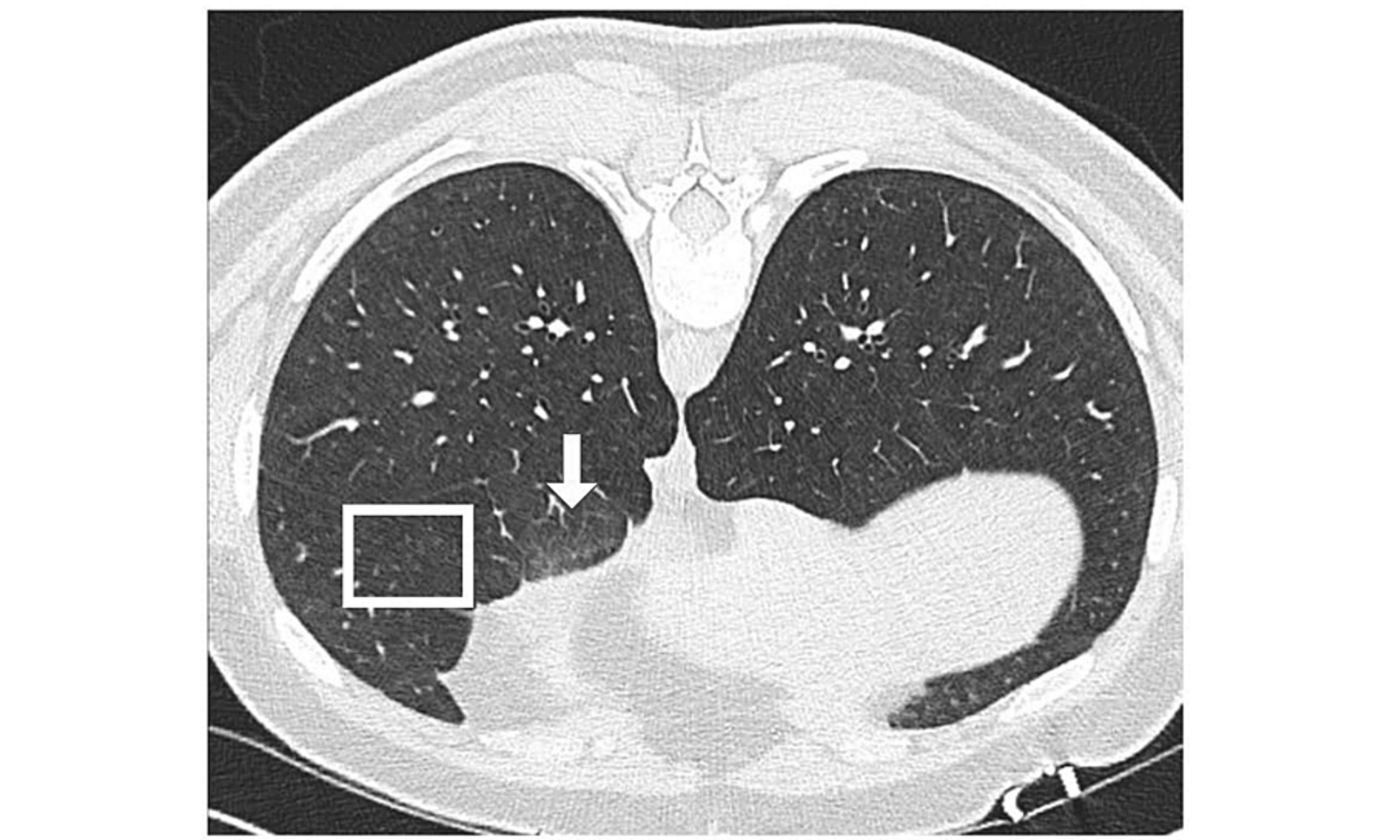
High-resolution CT chest demonstrating diffuse centrilobular ground-glass nodules (boxed) with some mosaic attenuation (arrow).
